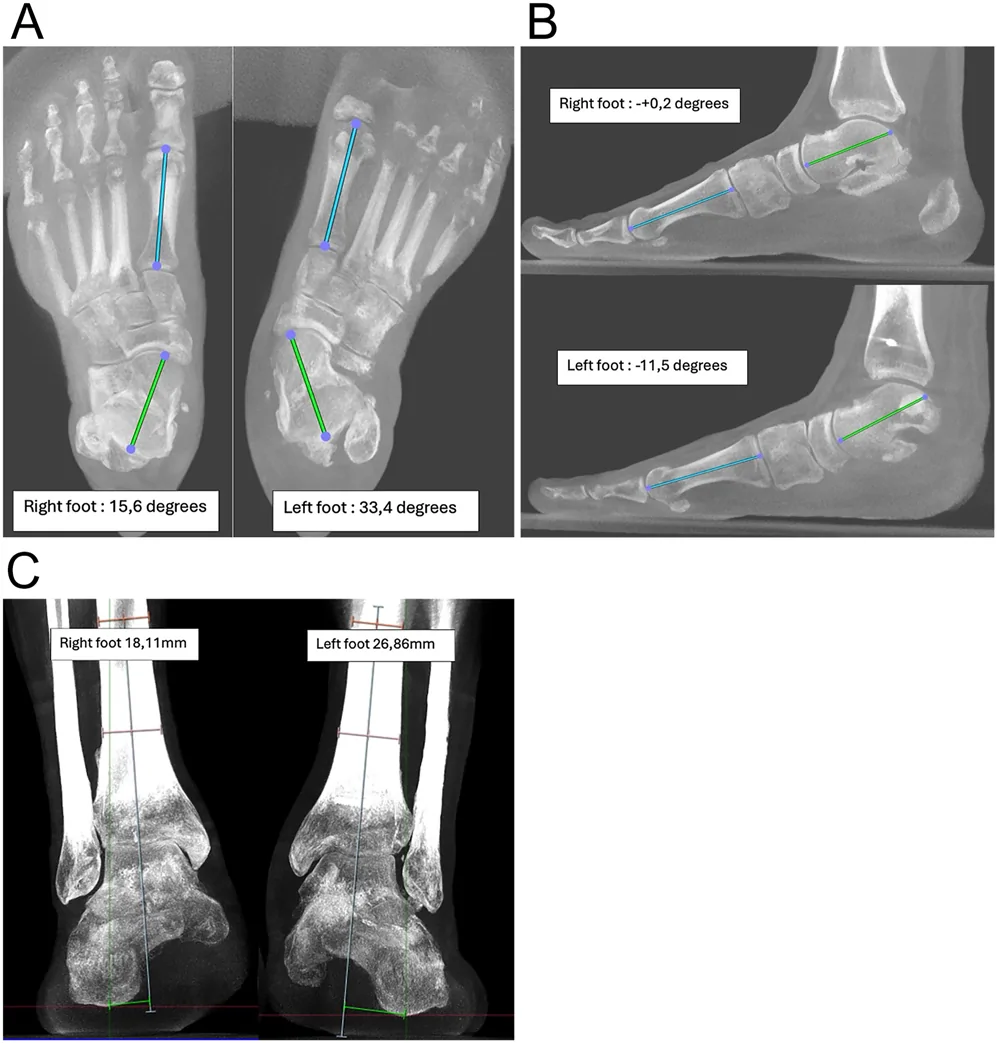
Weight-bearing computed tomography (WBCT) of the same patient shown in Fig. 3. The WBCT reveals segmental deformities marked by forefoot abduction and hindfoot valgus, consistent with a post-traumatic collapsing foot deformity on the left side. (A) Talus–first metatarsal angle – axial view (TFMA-A). (B) Talus–first metatarsal angle (TFMA-S). (C) Hindfoot moment arm (HMA).
